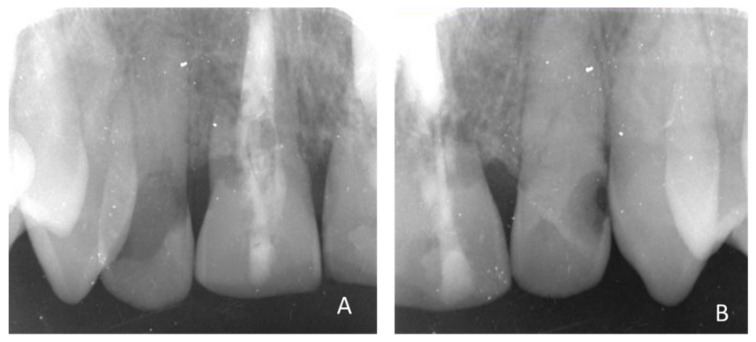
X-ray visualization of the invasive cervical resorptions of tooth 12 (A) and 22 (B).
